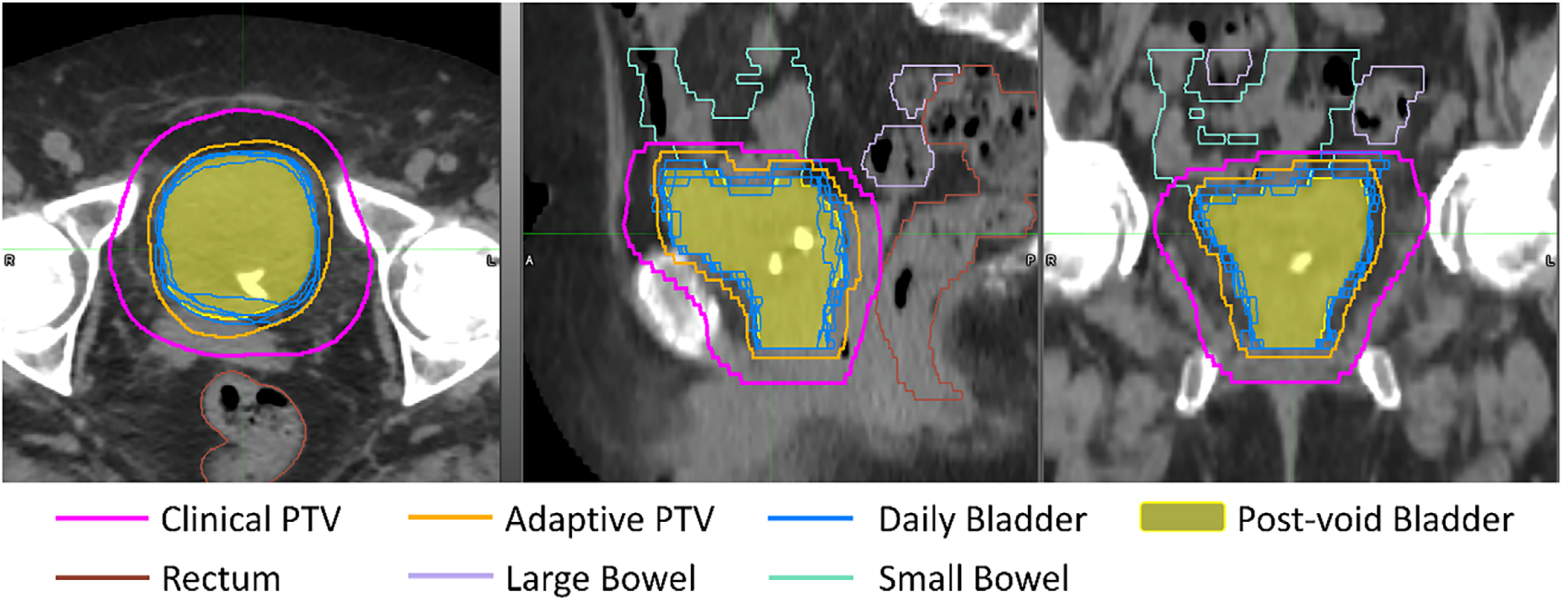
Comparison of the clinical PTV (purple) and the adaptive PTV (orange) on post‐void planning CT. The yellow color‐wash represents the (V_post_), the blue contours are the (V_daily_) from the first five fractions. Critical OARs include the rectum (brown), large bowel(violet), and small bowl (teal). OAR, organ at risks; PTV, planning target volume; (V_daily_), daily bladder volume; (V_post_), post‐void bladder volume.
